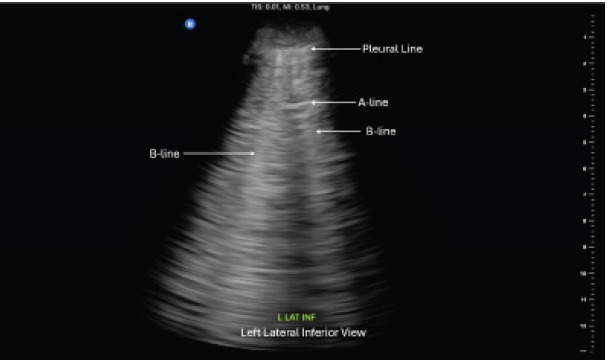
Point-of-care ultrasound of the left lung, in the left lateral inferior view, showing B-lines and partially obscured A-lines in a non-fatal drowning patient.
